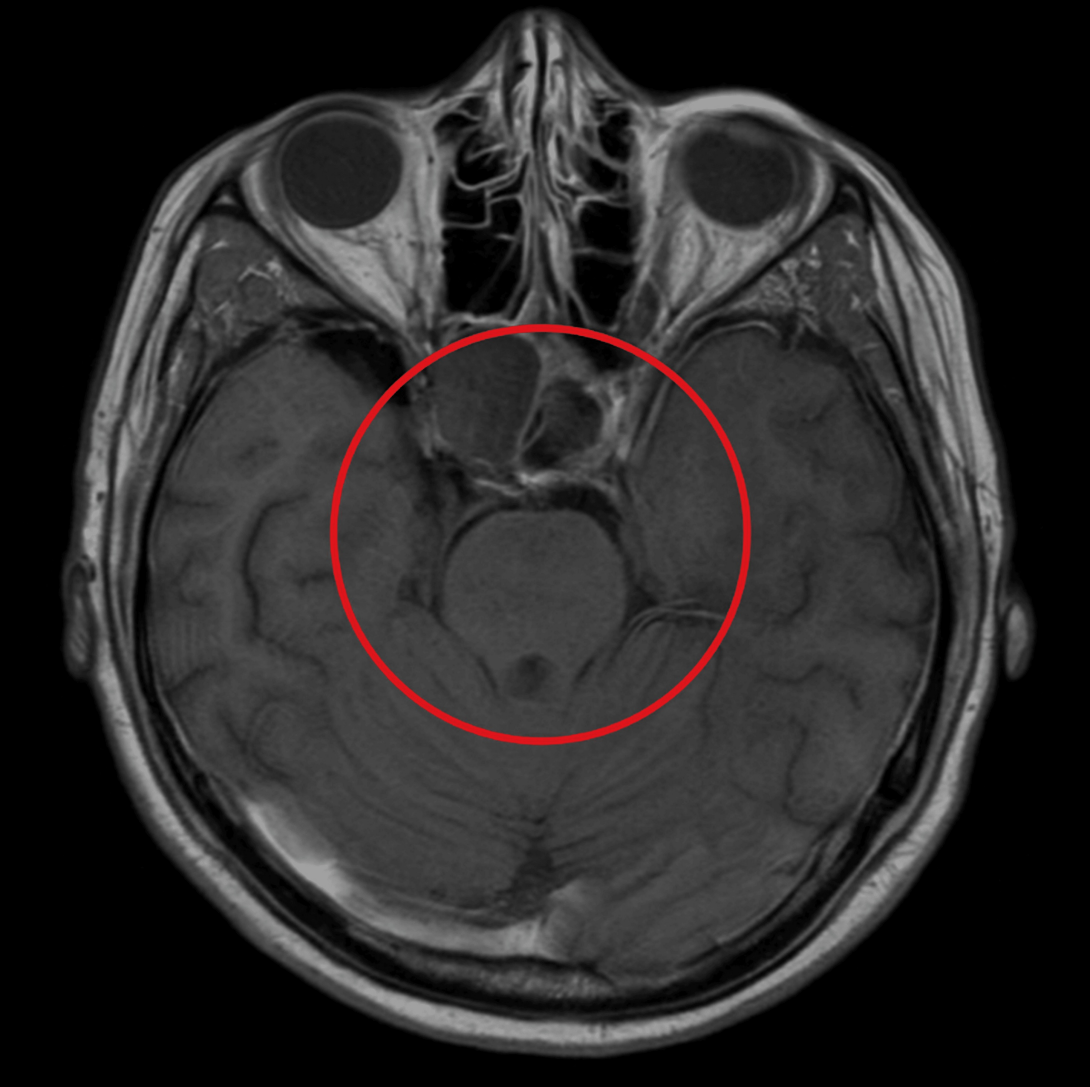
T1-weighted axial MRI showing a large, well-defined sellar and suprasellar mass (circled in red), consistent with an invasive pituitary macroadenoma. The lesion exerts a mass effect on the optic chiasm and extends laterally toward both cavernous sinuses
